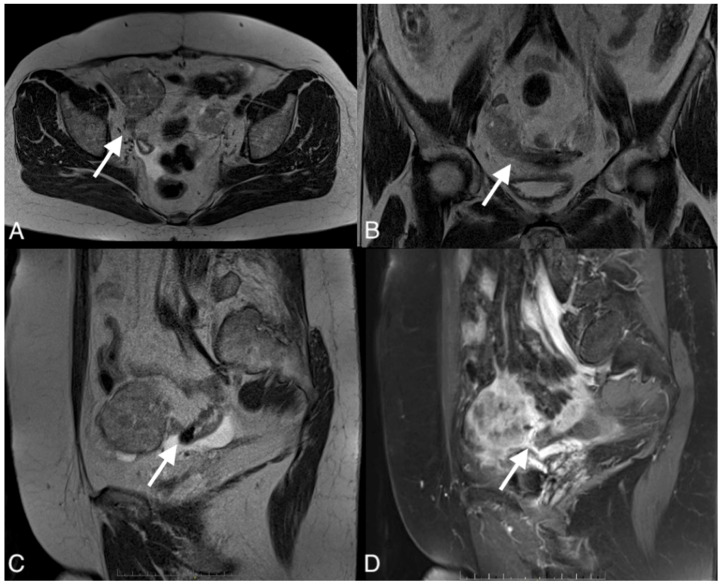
Images of a 67-year-old woman with a recurrent high grade serous ovarian cancer. MRI T1-weighted post-contrast image obtained in the axial plane (A), coronal plane (B), sagital plane (C), and T1-weighted image post-contrast (D) show large pelvic tumor and fistula (arrows) between the tumor and sigmoid colon.
